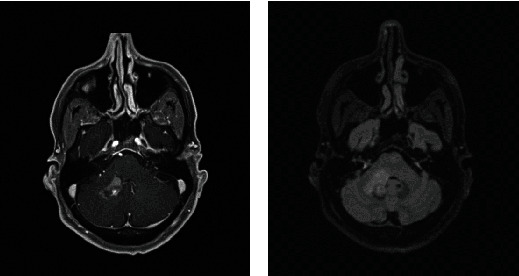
Masslike T2/FLAIR hyperintense enhancing lesion centered in the right brachium pontis extending into the dorsal pons and medulla with extension to the right trigeminal nerve root entry zone with additional multiple nonenhancing white matter lesions in the supratentorial and infratentorial brain. Radiographic differential diagnosis: tumefactive demyelination, primary CNS neoplasm, and leptomeningeal enhancement.
